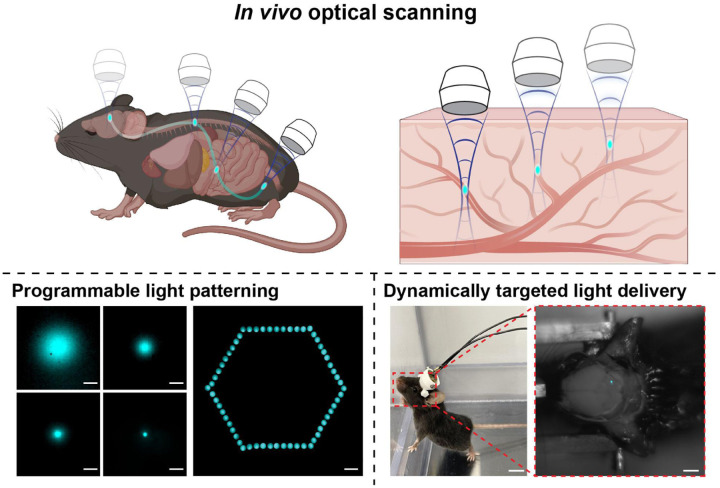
Optical scanning of biological systems via an ultrasound-mediated light source. The combination of relocatable FUS and the circulating mechanoluminescent nanotransducers (MLNTs) allows for dynamic optical scanning of the complex biological systems via the endogenous vascular network. This platform supports programmable light patterning (scale bars, 1 mm) and dynamically targeted light delivery (scale bars represent 1 cm and 5 mm, respectively).
